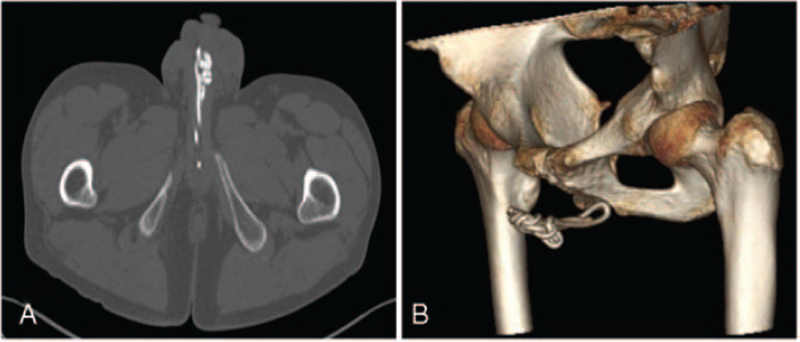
CT imaging of the sacrum. A. Axial image of CT of male genital organ. B. Volume rendering of CT.
